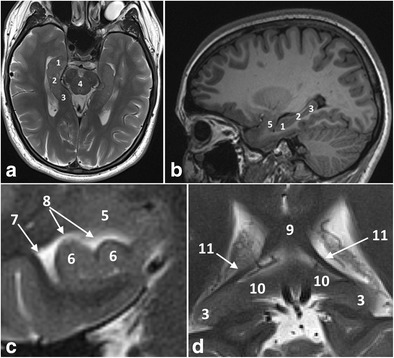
Anatomy of the hippocampal formation on 3-T axial T2 (a) and sagittal 3D-MPRAGE images (b). Zoomed-in 3-T coronal T2-weighted images at the level of the hippocampal head (c) and the hippocampal tail (d). The hippocampal body is shown in detail in Fig. 2. *1 = hippocampal head, 2 = hippocampal body, 3 = hippocampal tail, 4 = mesencephalon, 5 = amygdala, 6 = hippocampal digitations, 7 = temporal horn of the lateral ventricle, 8 = uncal recess of the lateral ventricle, 9 = splenium of the corpus callosum, 10 = subsplenial gyri, 11 = crura of the fornices.* To easily recognize the different portions of the hippocampus, we can use the mesencephalon (4). The head (1) is located in front of the mesencephalon, the body (2) can be found at the level of the mesencephalon and the tail (3) is posterior to the mesencephalon. The hippocampal head is the only portion of the hippocampus not covered by the choroid plexus (7). The hippocampal head is separated from the amygdala (5) by the uncal recess of the lateral ventricle (7) and is characterized by small digitations separated by small sulci, the digitationes hippocampi (6). At the level of the hippocampal tail, the fimbriae continue posteriorly as the crux of the fornix (11) that slants upwards towards the splenium of the corpus callosum (9) and the hippocampal tail continues as the subsplenial gyri (10).
